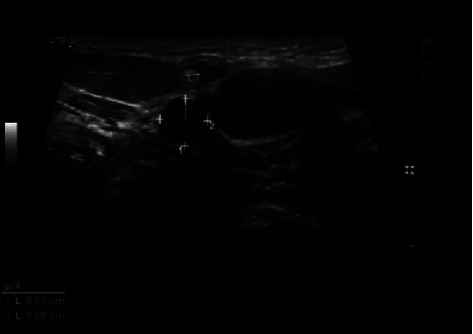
Neck ultrasound of the left lateral aspect of the neck shows a 7 × 7 mm rounded lymph node, with loss of fatty hilum, located medially to the internal jugular vein.
